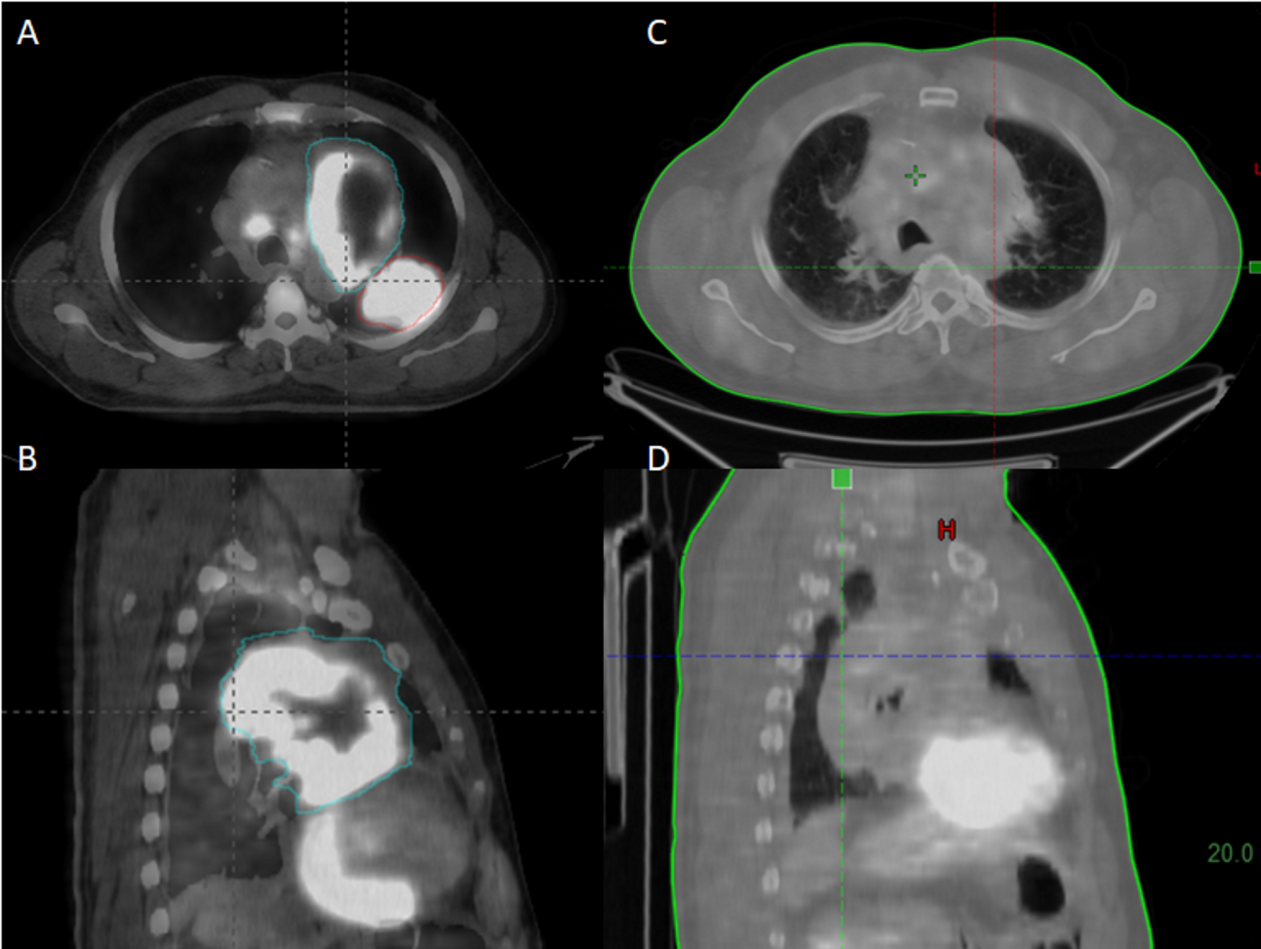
Complete response as per standardized uptake value (SUV) A 62-year-old male with advanced adenocarcinoma of the left lung. Figures 4A-4B: axial and sagittal views (soft tissue windows) of fluorodeoxyglucose (FDG) positron emission tomography-computed tomography (PET-CT) before LRT demonstrating two masses in the left lung active on PET and pre-tracheal adenopathy. Figures 4C-4D: corresponding axial and sagittal views (mediastinum windows) of follow-up FDG PET-CT 22 months after treatment showing a persistent mediastinal mass on corresponding CT but no significant uptake in the mediastinal mass or in adenopathy. The posterior mass in the left lung was no longer evident.
